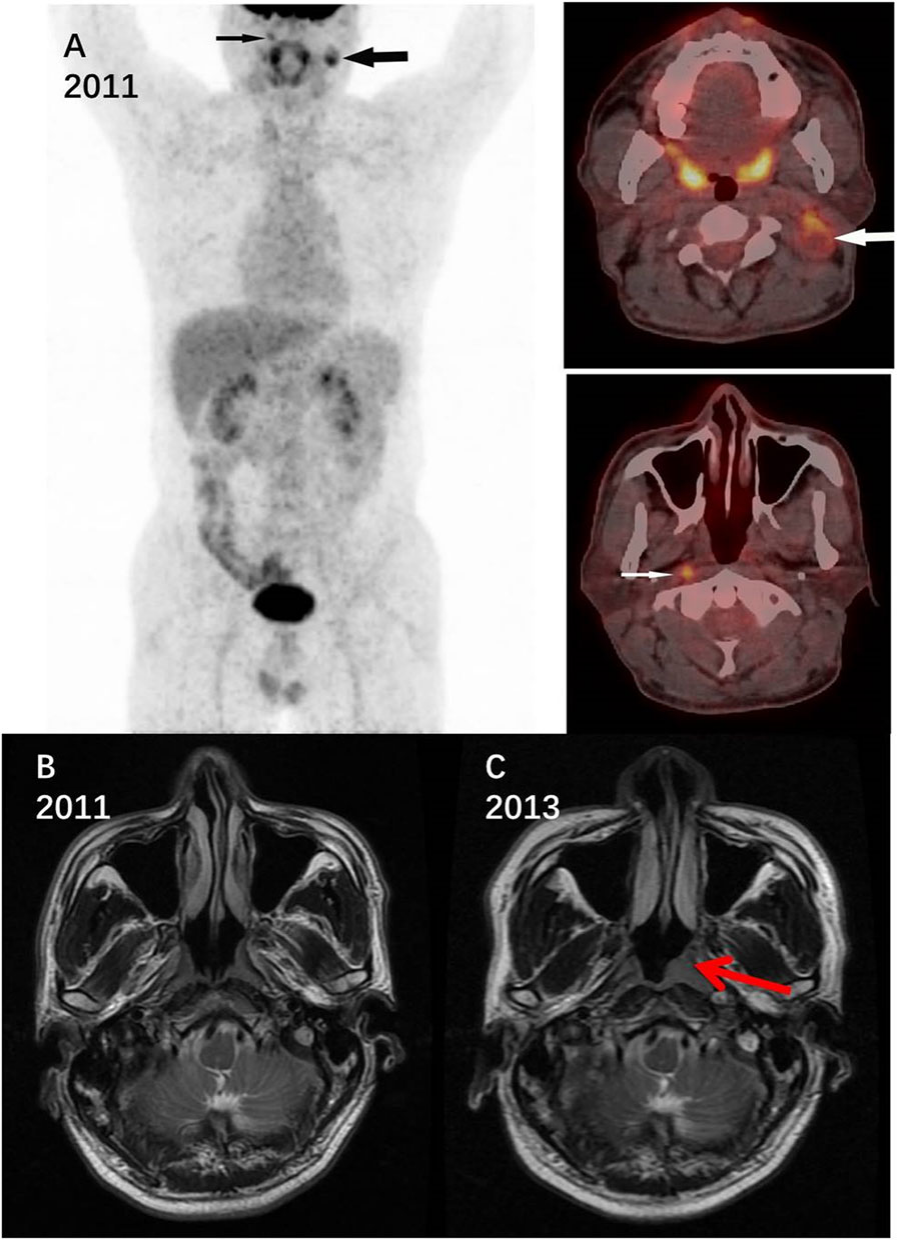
Images of a clinical CUP patient with cervical lymph node metastases. Nasopharynx endoscopy was negative in 2011, with the primary lesion emergent in 2013. The primary cancer was finally pathologically confirmed to be nasopharyngeal cancer, demonstrating the value of SLN theory in tracking the primary. A: Image of 18F-FDG PET-CT scan in 2011 showing only cervical lymph node metastases (black and white arrow) and was otherwise normal. B: Magnetic resonance imaging of nasopharynx in 2011 with no suspicious lesion. C: Magnetic resonance imaging of nasopharynx in 2013 with a suspicious lesion (red arrow), which was later confirmed by biopsy and pathological data
